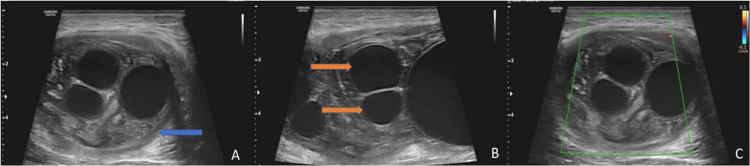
Ultrasonography (USG) images of the right thigh showing hydatid cyst. (A) Axial and (B) longitudinal sections revealing a large mother cyst(blue arrow) with a heterogeneous matrix and multiple smaller daughter cysts within (orange arrows). (C) The axial section of color Doppler USG revealing no significant vascularity within the lesion.
